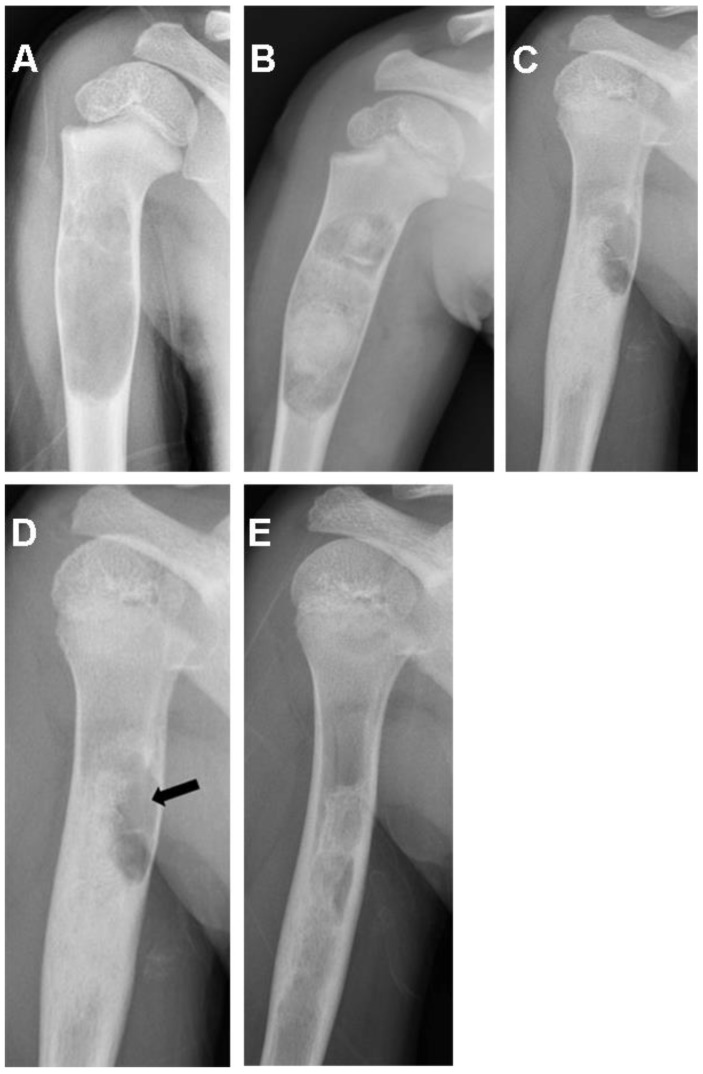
(A) Preoperative radiograph of a unicameral bone cyst of the humerus. (B) Immediate postoperative radiograph after curettage and bone graft filling <90% of the cavity. (C,D) Postoperative 6- and 12-month follow-up radiographs showing radiolucent area <50% (arrow) of the bone diameter (Neer II). (E) Twenty-four-month follow-up radiograph showing cortical thickening, bone remodeling, and small intramedullary cystic remnants.
